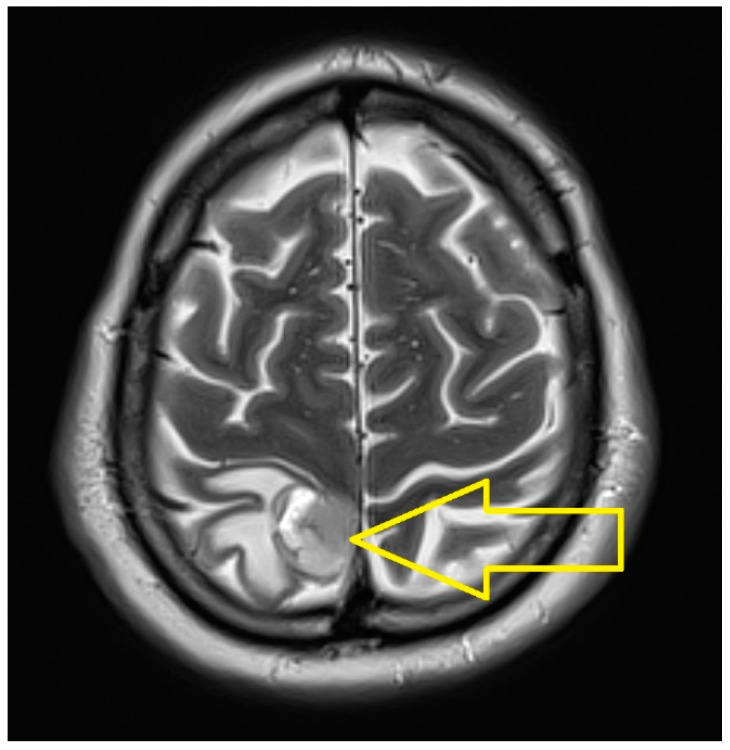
The cranial MRI (March 2023): Right parietal nodule that is compatible on imaging with a secondary hematogenous mass with intralesional bleeding.
